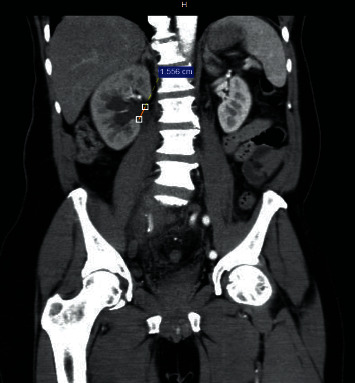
CT-IVU showing the right sided hydroureteronephrosis
